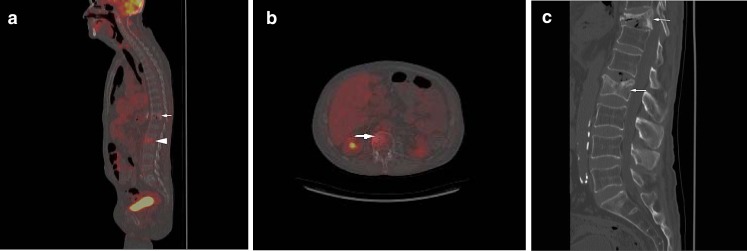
a False-negative compression fracture on FDG-PET in a patient with metastatic esophageal cancer. Sagittal fused FDG-PET/CT image demonstrates mild to moderately increased radiotracer uptake in the L2 compression fracture (*arrow*; SUV = 2.5) that was thought to represent a benign compression fracture but was found to be malignant on subsequent biopsy. Note the mildly increased radiotracer uptake in the T11 compression fracture (*arrowhead*), which was thought to represent a benign fracture. b Axial fused FDG-PET/CT image demonstrates increased radiotracer uptake in the L2 vertebral body (*arrow*). c Sagittal CT image demonstrates L2 and T11 compression fractures (*arrows*), for which malignancy was indeterminate
